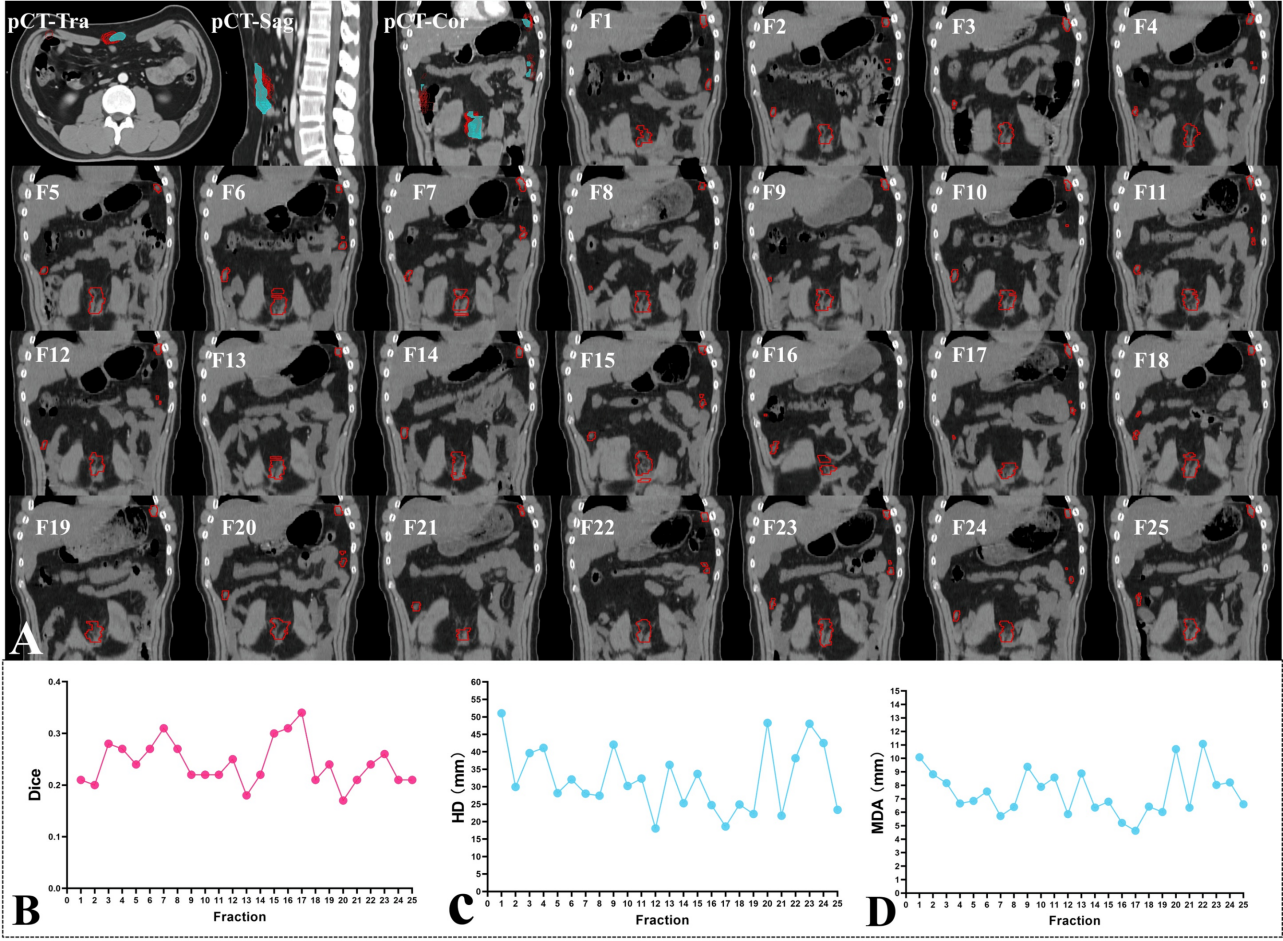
Changes in the position and shape of the GTV during the 25 ART fractions and the corresponding variations in DSC, HD, and MDA In Figure 2A's pCT-Tra, pCT-Sag, and pCT-Cor images, the blue region represents the original GTV while the red line represents all the re-delineated GTVs delineated from daily FBCT (F1-F25) acquired over 25 ART fractions. Figures 2B-2D show the Dice, HD, and MDA variations of the GTV in 25 fractions (compared to the GTV in the planning CT). *Abbreviations: *GTV: Gross tumor volume; ART: Adaptive radiotherapy; DSC: Dice similarity coefficient; HD: Hausdorff distance; MDA: Mean distance to agreement. pCT: Planning computed tomography; Tra: Transverse view; Sag: Sagittal view; Cor: Coronal view; F: Fraction; FBCT: Fan beam computed tomography
